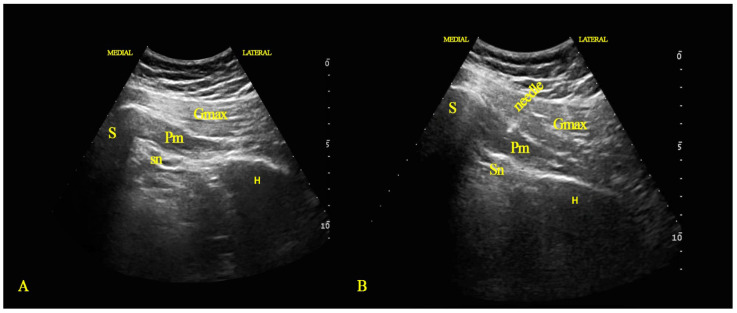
Ultrasound scan (A) and ultrasound-guided injection (B) for piriformis syndrome. S: sacrum; H: hip bone; Gmax: gluteus maximus muscle; Pm: piriformis muscle; Sn: sciatic nerve.
